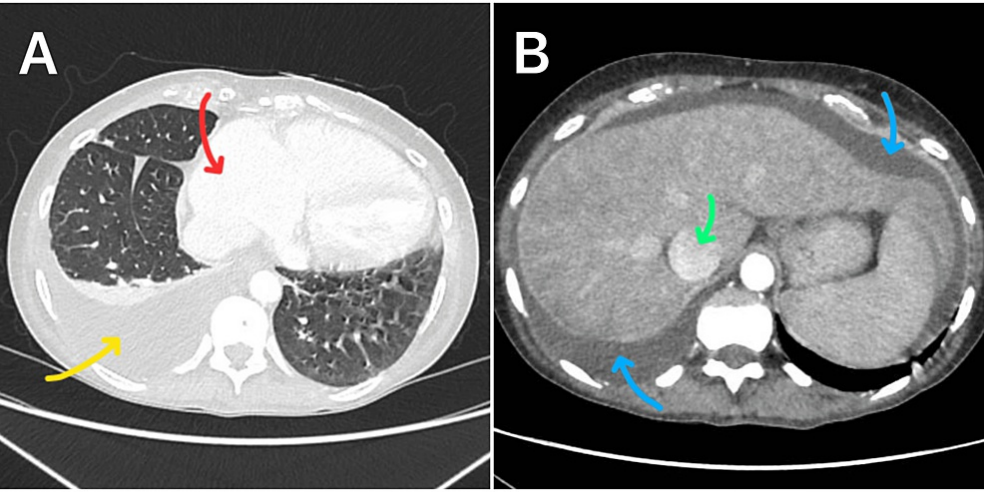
Initial CT scan of the chest (A) and abdomen (B) The images show a moderate right-sided pleural effusion (yellow arrow), cardiomegaly with bilateral atrial dilatation (red arrow), moderate ascites (blue arrows), and hepatomegaly with prominent dilatation of the inferior vena cava (green arrow) without any evidence of liver diseases such as cirrhosis, hepatocellular carcinoma, or Budd-Chiari syndrome, making the diagnosis of passive hepatic congestion more likely CT: computed tomography
